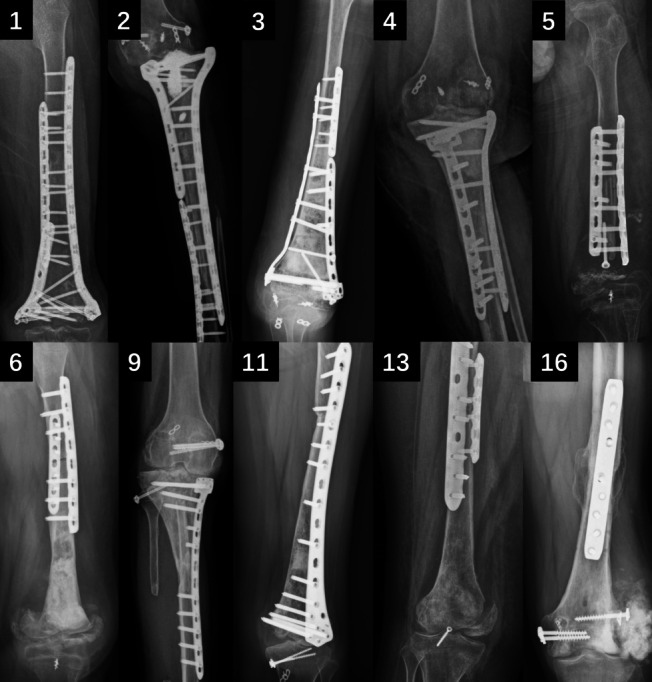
X-rays of cases of joint dislocation or fracture. Cases 1, 2, 3, 4, 5, 6, 9, 11, 13, and 16 all showed varying degrees of fractures at the joint end of the inactivated bone. Cases 2, 3, 5, 6, 9, 11, 13, and 16 are accompanied by varying degrees of joint dislocation. Except for cases 6 and 13, all of the above cases achieved bone healing at the shaft site of the inactivated bone
